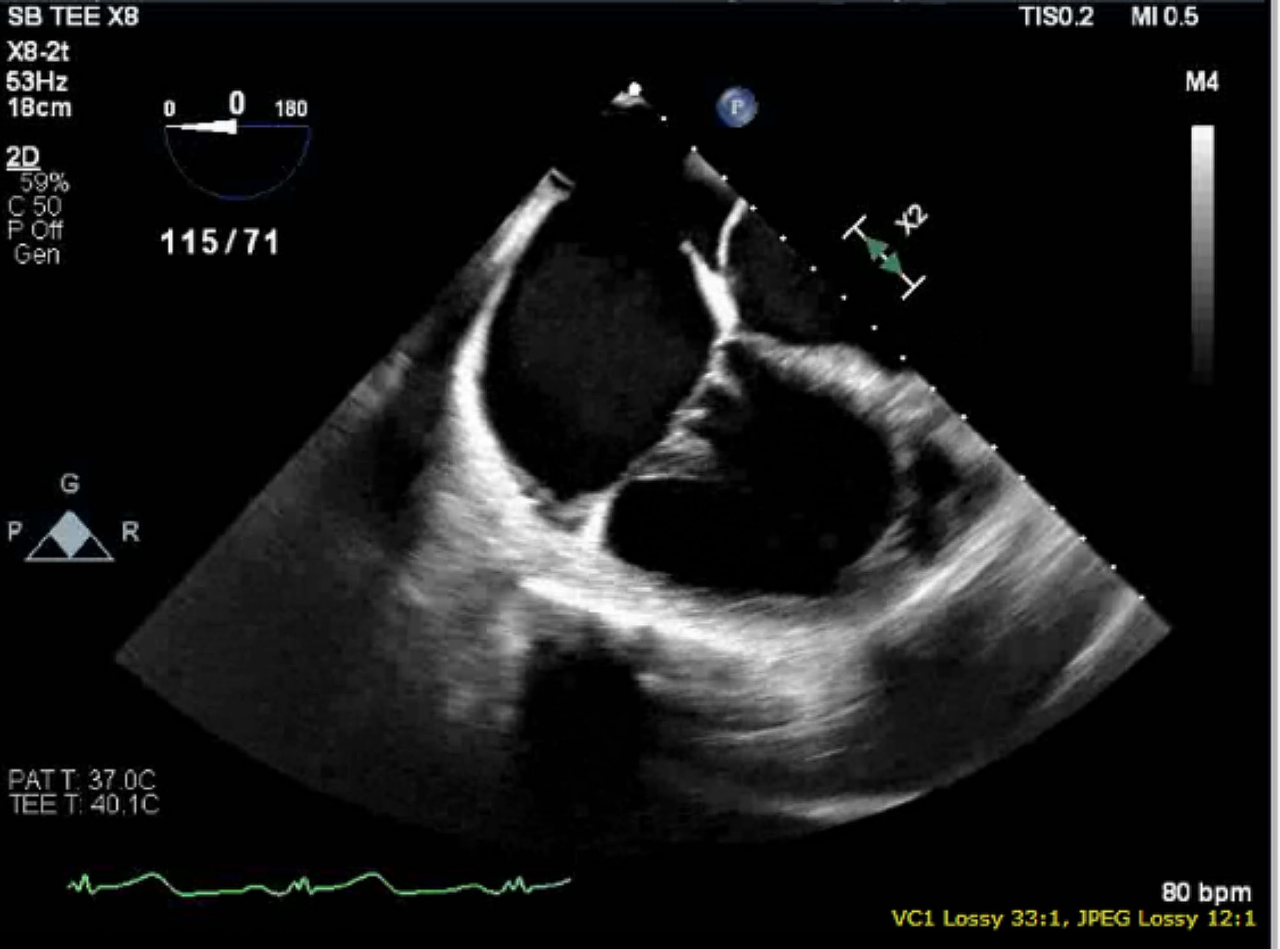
Pre-operative transesophageal echocardiogram demonstrates the presence of an atrial septal defect
